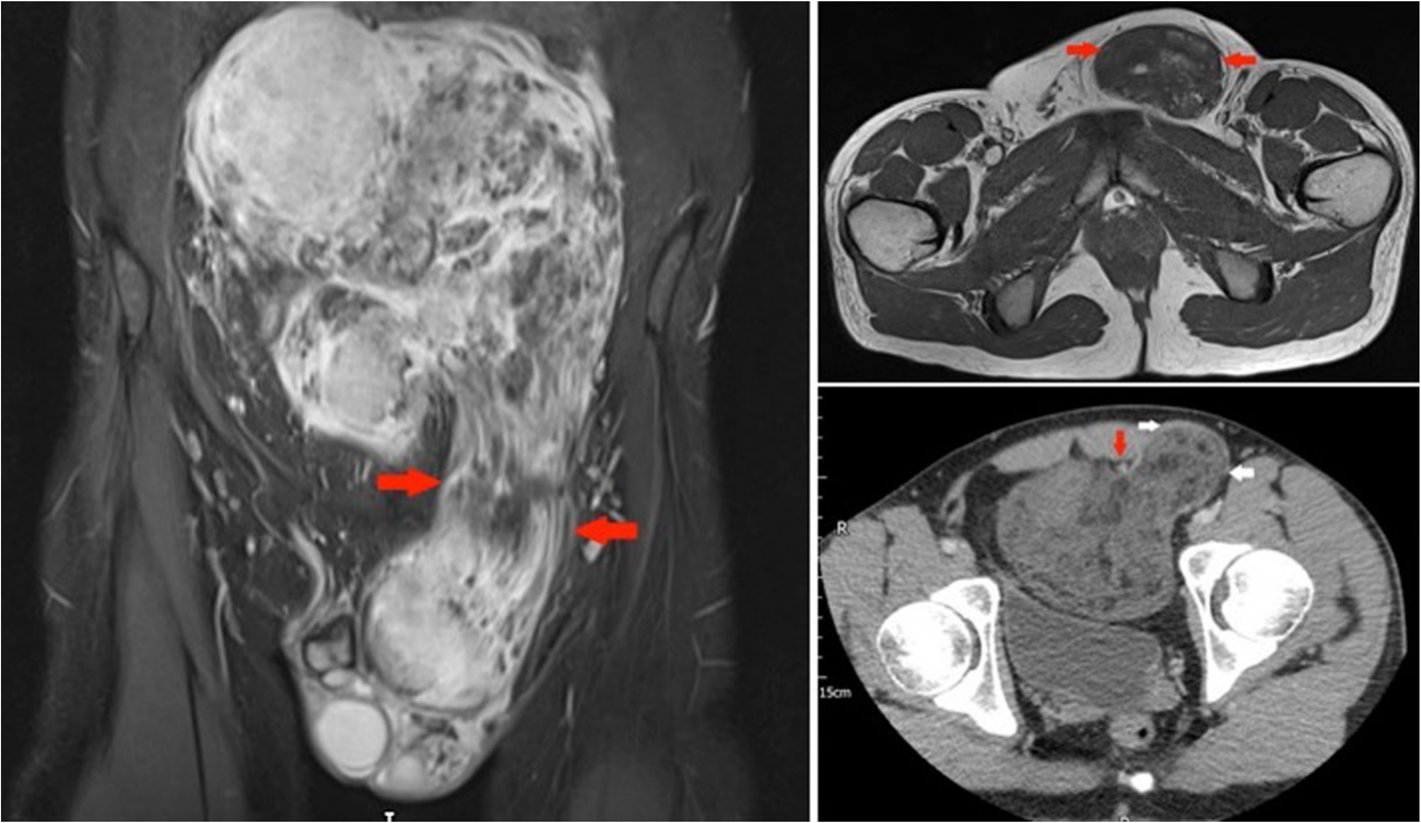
Left panel: Coronal T1 TIRM-weighted MR image of the abdomen and pelvis: large intra-abdominal mass with areas of fat suppression suggesting a fat containing tumour. The tumour is seen to herniate through the left inguinal canal (red arrows). Right upper panel: Axial T1-weighted MR image of the pelvis: A large left indirect inguinal hernia is observed (red arrows). On T1-weighted imaging fat content is identified which would suggest liposarcoma. Right lower panel: Axial contrast-enhanced CT of the abdomen and pelvis: a large intra-pelvic lesion is observed herniating through a groin defect (white arrows) lateral to the inferior epigastric vessels (red arrow). It appears to be displacing and compressing the bladder posteriorly
